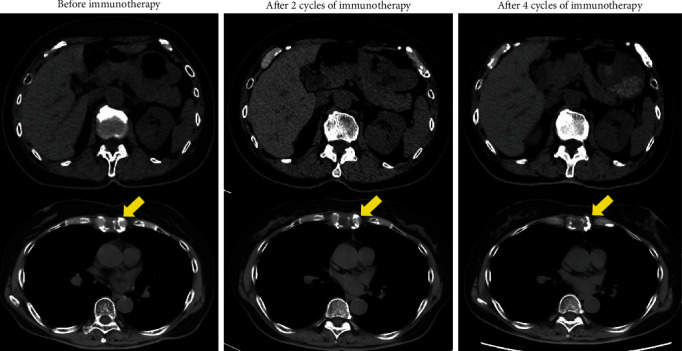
Computed tomography (CT) findings in Case 1. Abdominal CT showed no change in the right primary renal tumor or bone metastasis after four cycles of immunotherapy.
